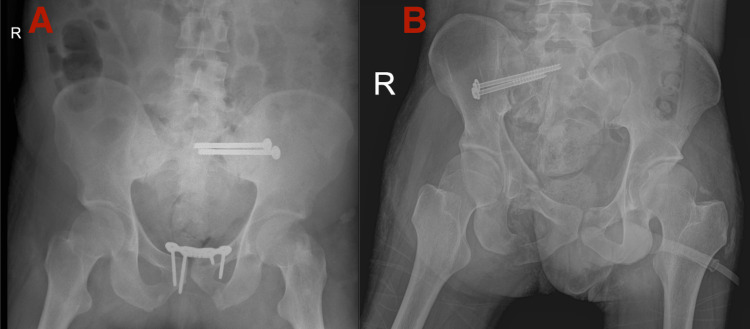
Postoperative radiograph showing left sacral ala screw fixation and reduced symphysis pubis disruption for the first patient (A) and right sacroiliac screw fixation for second patient (B) following osteosynthesis.
